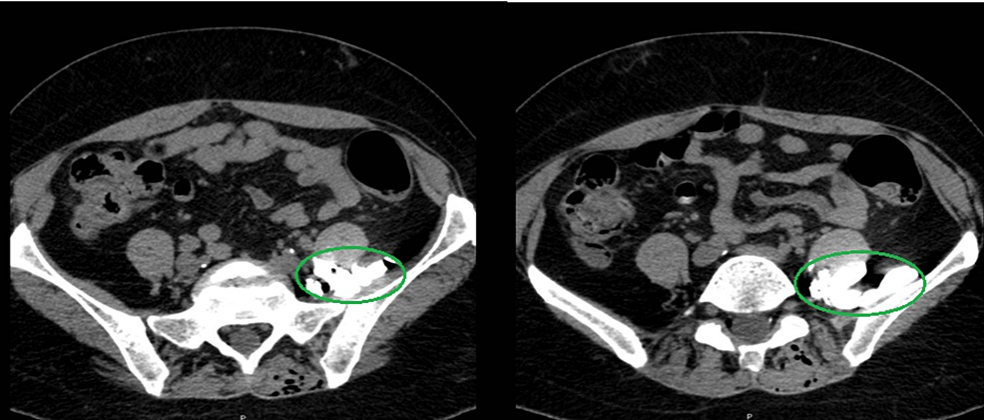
Transverse CT scan after administration of radiopaque contrast through the epidural catheter in case 1 Circles show enhancement in the left psoas major muscle after epidural contrast administration.
